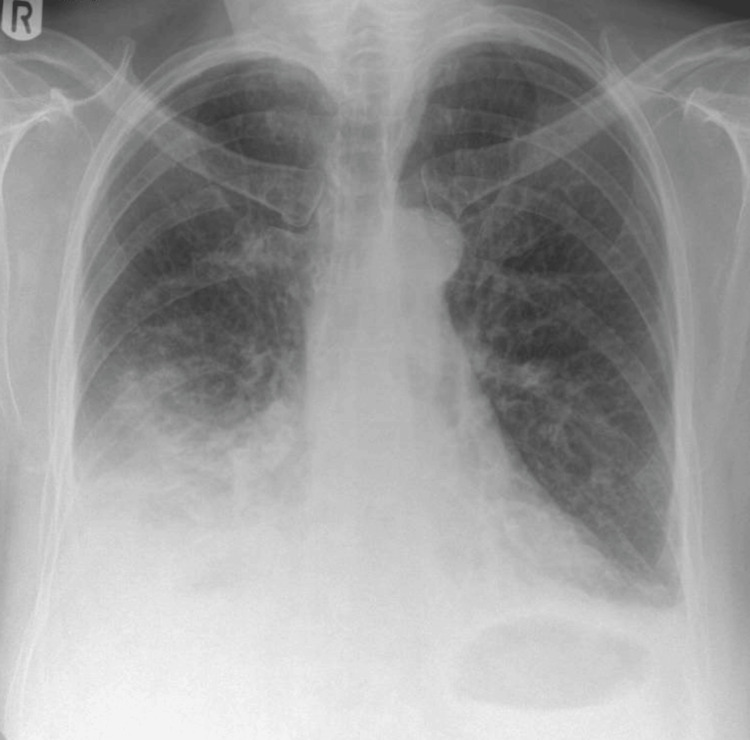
Chest radiograph demonstrating right middle and lower lobe consolidation Chest radiograph obtained at admission demonstrating dense consolidation involving the right middle and lower lobes, consistent with severe community-acquired pneumonia at initial presentation.
